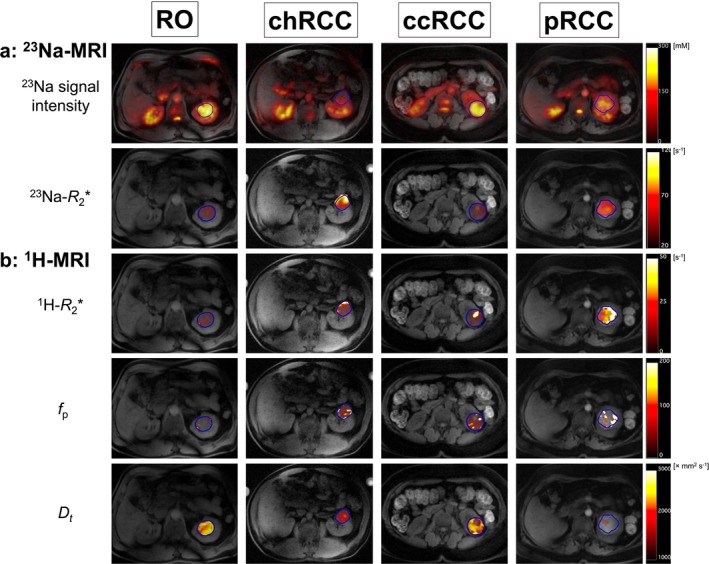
Representative parameter maps derived from: (a) ^23^Na‐MRI, including ^23^Na signal intensity and ^23^Na‐*R*
